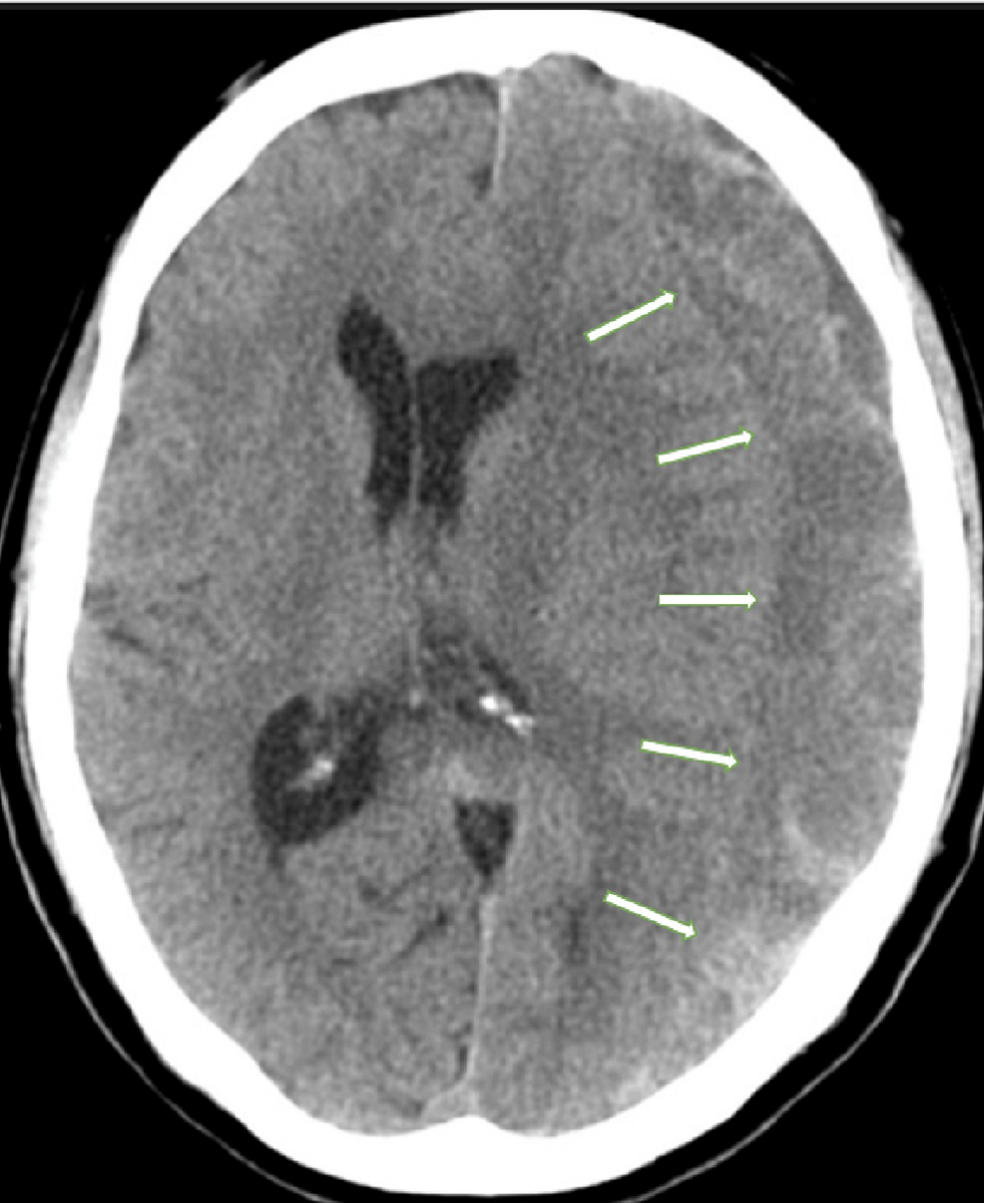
Brain computed tomographic findings A left chronic subdural hematoma is identified. The hematoma shows a hetero-density signal (arrows).
